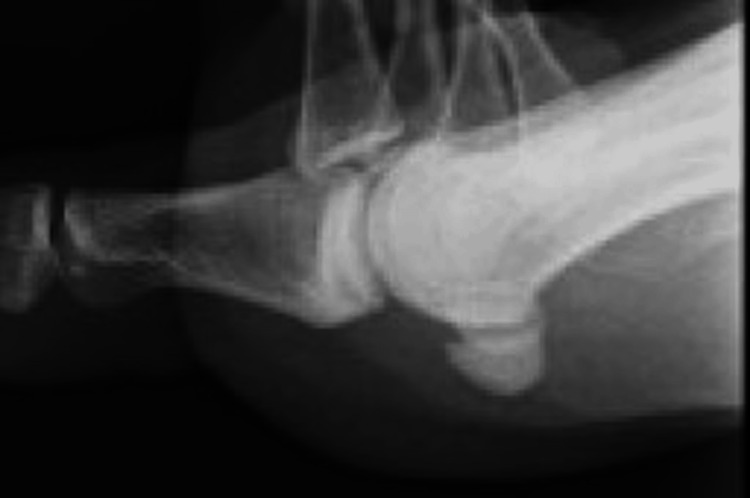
A final X-ray Calcification was no longer visible on the final X-ray.
